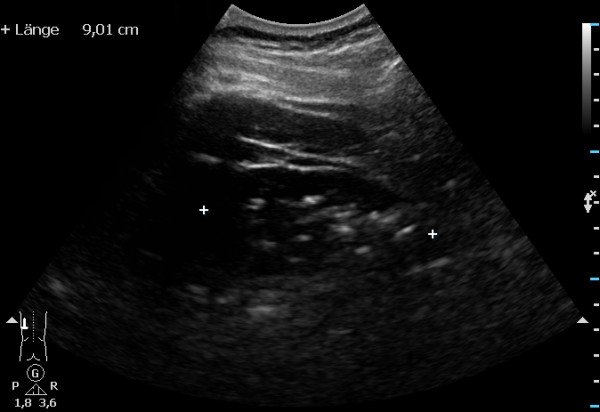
Very poor quality of ultrasound image of the kidney. The evaluation of reliable measurements of the kidney was unsuccessful due to severe gas artifacts.
